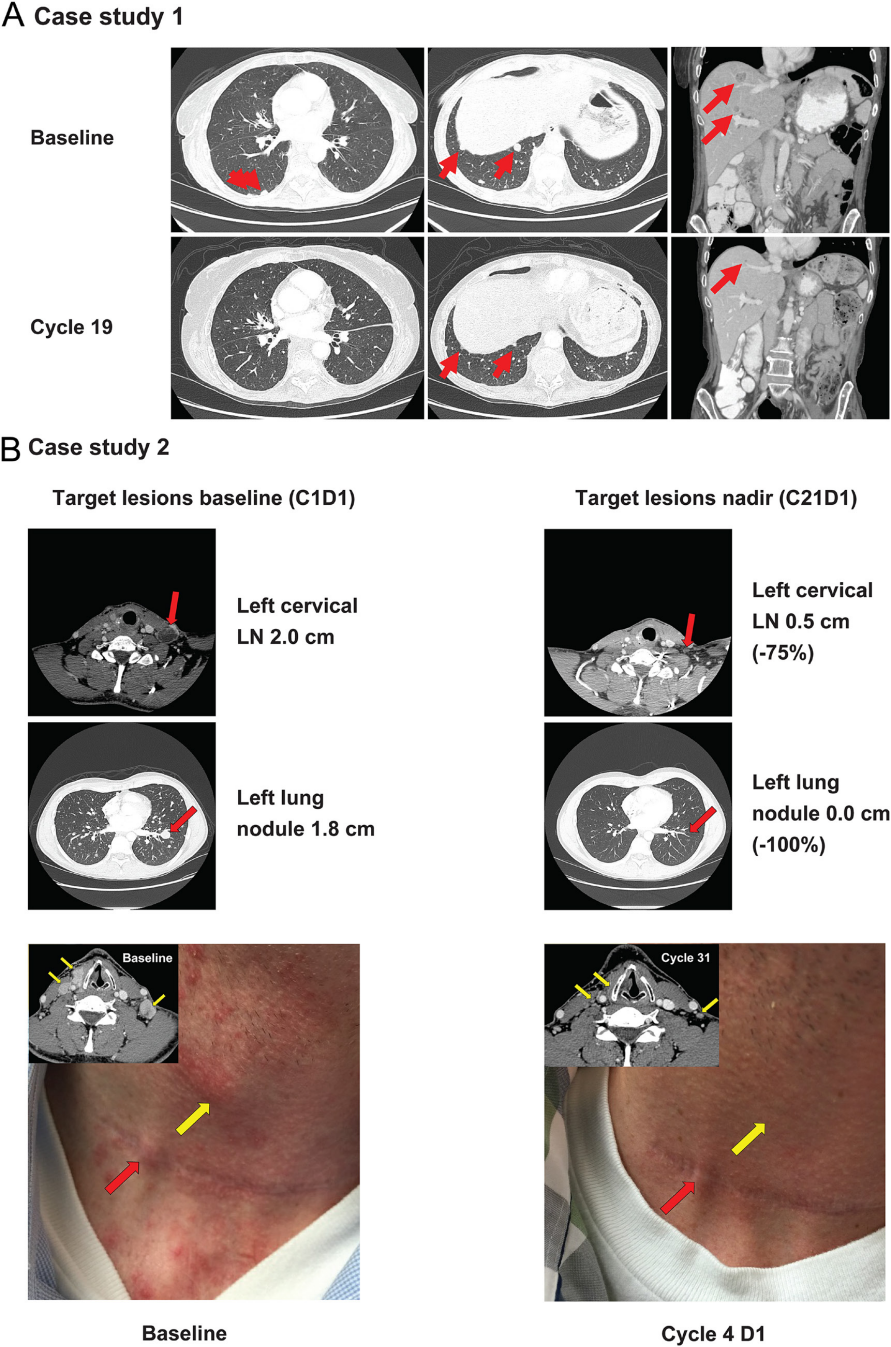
Case studies of patients with advanced TRK fusion-positive thyroid carcinoma treated with larotrectinib. Contrast-enhanced CT images demonstrating response in target and non-target lesions. (A) An adult female patient was diagnosed with PDTC with an *ETV6-NTRK3* gene fusion. The red arrows indicate lung and liver metastases that responded to therapy. (B) An adult male patient was diagnosed with PTC with an *ETV6-NTRK3* gene fusion. The red arrows indicate target lesions, and the yellow arrows identify non-target lesions. (C) A paediatric male patient was diagnosed with PTC with an *IRF2BP2-NTRK1* gene fusion and a complete response in a lung target lesion (yellow arrows). LN, lymph node; *NTRK*, neurotrophic tyrosine receptor kinase; PDTC, poorly differentiated thyroid carcinoma; PTC, papillary thyroid carcinoma; TRK, tropomyosin receptor kinase. A full colour version of this figure is available at https://doi.org/10.1530/EJE-21-1259.
